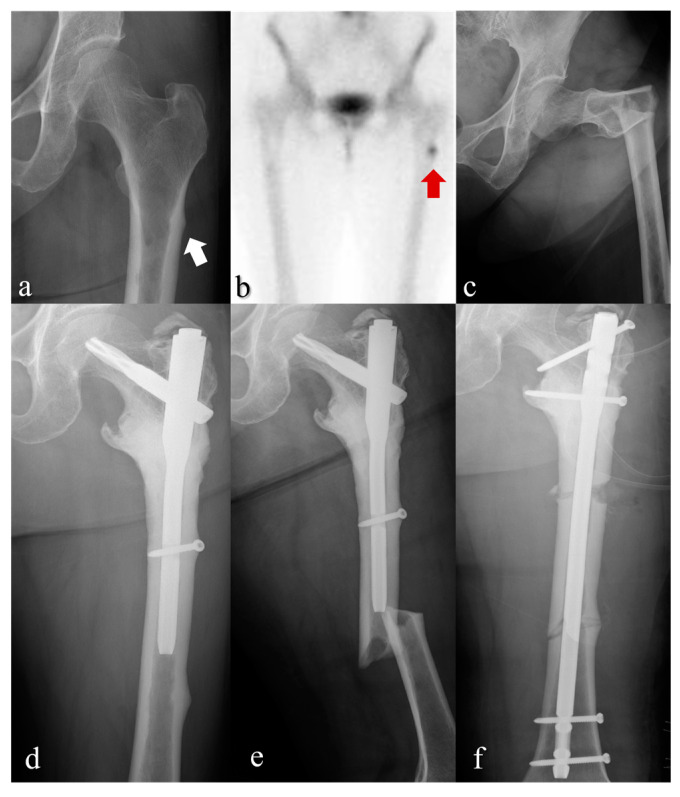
Anteroposterior view radiographs of the left femur (a,c–f) and a bone scan image (b) of two consecutive AFFs (arrows) occurred in a patient treated with high-dose bisphosphonates. (AFF: atypical femoral fracture).
